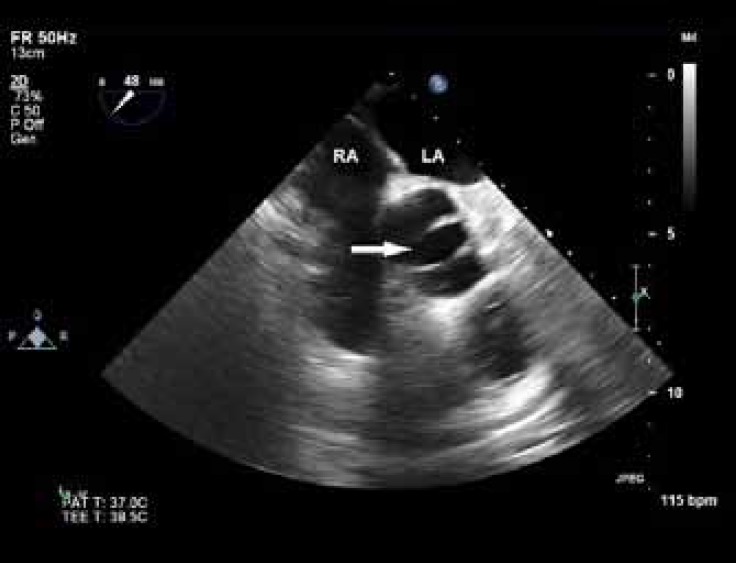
Aortic short-axis view in transesophageal echocardiography, showing the bicuspid aortic valve (arrow): a rare and interesting finding in Ebstein’s anomaly
